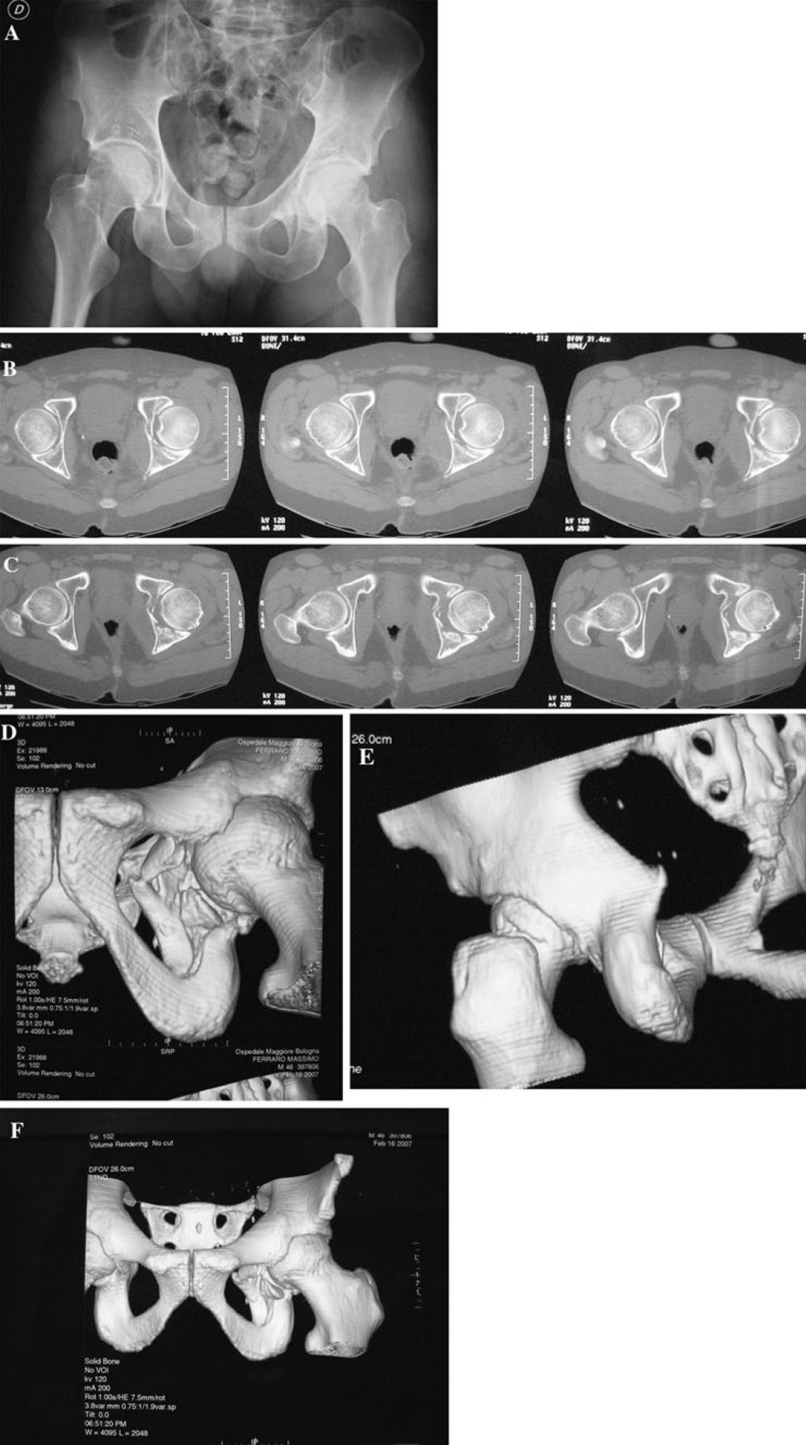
a X-ray of the pelvis showing fracture of upper part of the ischium in the lateral part of the obturator foramen. b, c Computed tomography (CT) showing the impacted fragment of the articular surface and comminution of the*lamina quadrilatera*. d–f 3D CT highlighting the well-impacted articular fragment and involvement of the*lamina quadrilatera*. The anterior and posterior columns are intact
